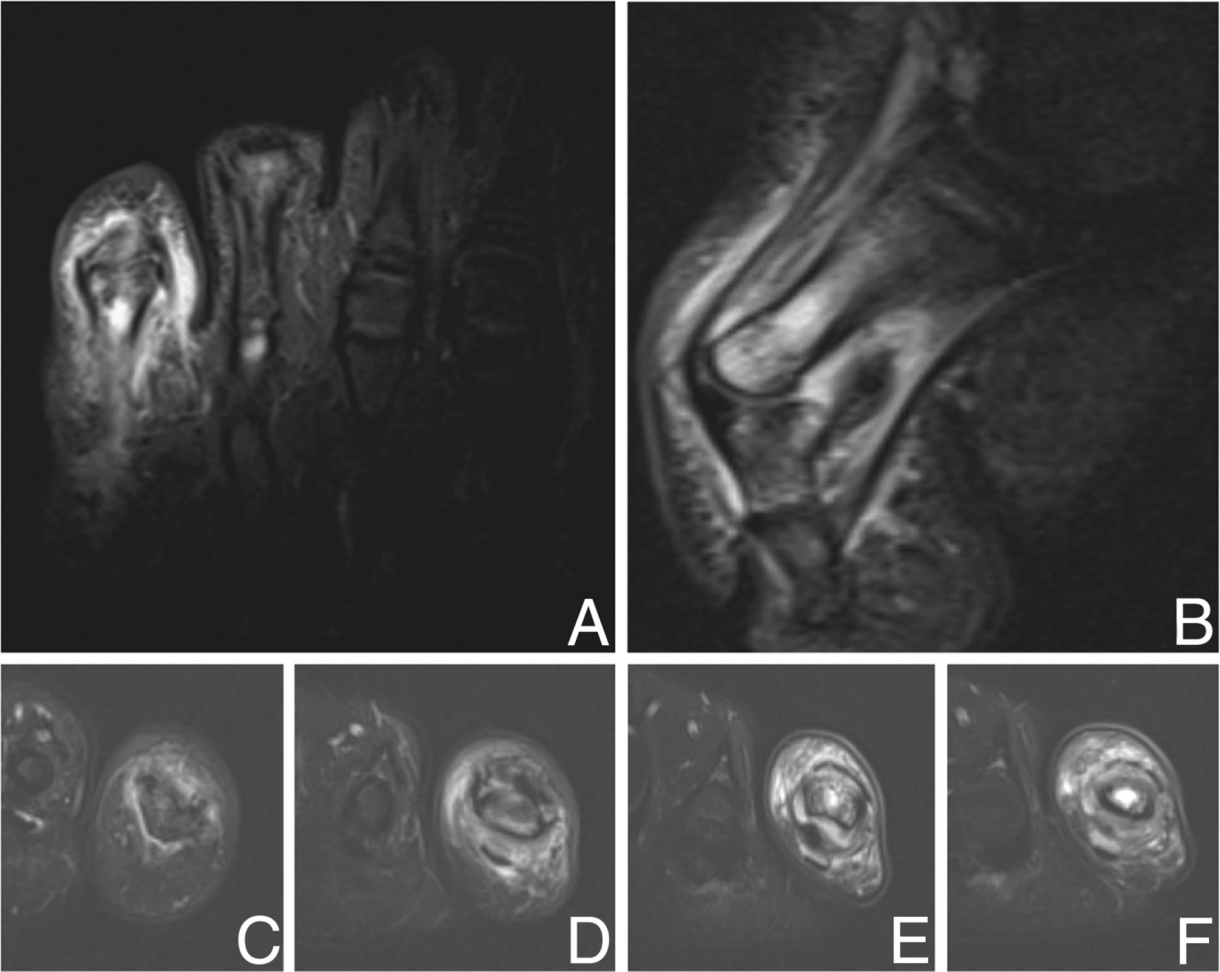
Magnetic resonance imaging evaluation of dactylitis. A. Coronal short tau inversion recovery (STIR), B. sagittal T2 with fat suppression, and C-F. axial T2 with fat suppression of the left fifth toe, proximal to distal sequences, demonstrating increased signal and gadolinium enhancement at the local entheses without associated tendonitis or synovitis.
